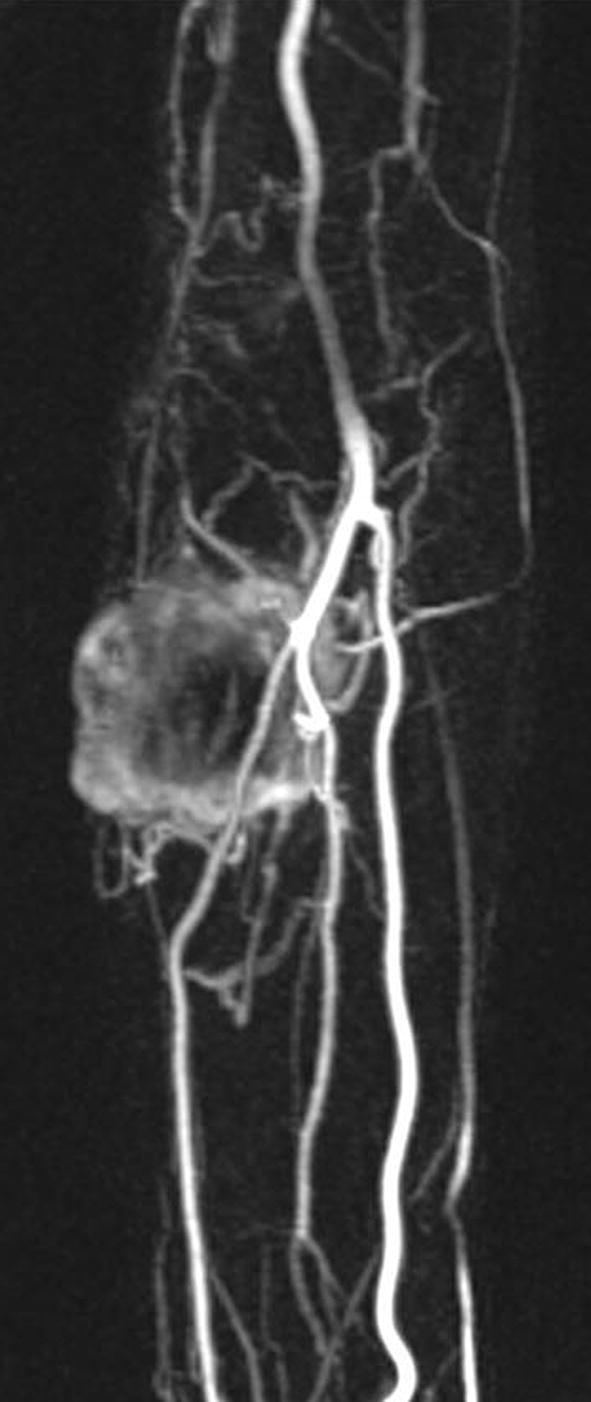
Maximum intensity projections with dynamic contrast enhanced magnetic resonance imaging (DCE-MRI) showing high vascularization in the arterial phase
